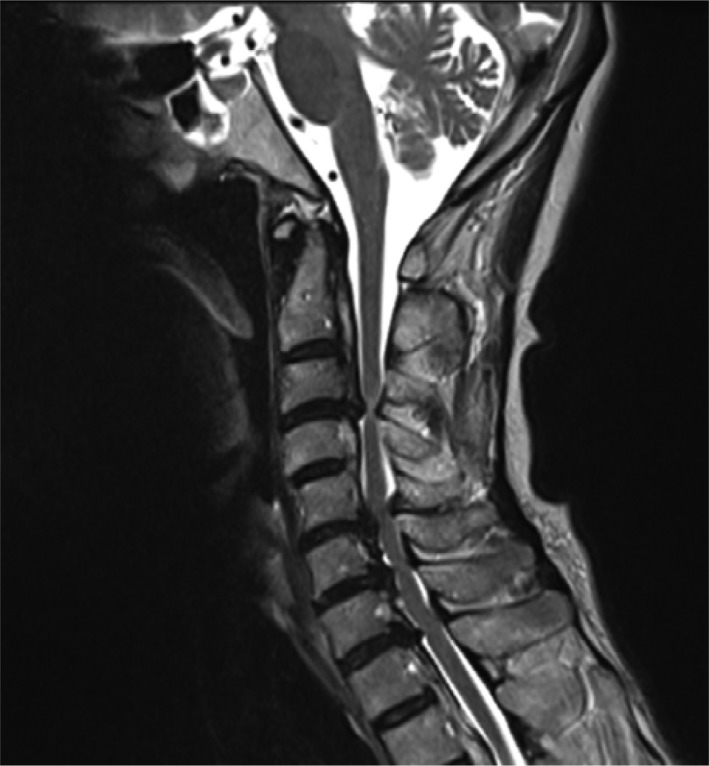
Sagittal T2‐weighted magnetic resonance image (MRI) of the cervical spine before laminoplasty. Sagittal T2‐weighted MRI revealed atrophy of the spinal cord and an intramedullary T2‐weighted high‐intensity area in the spinal cord at C3/4 level. Mild stenosis is also seen at C5/6 and C6/7, but there is no high‐intensity area
